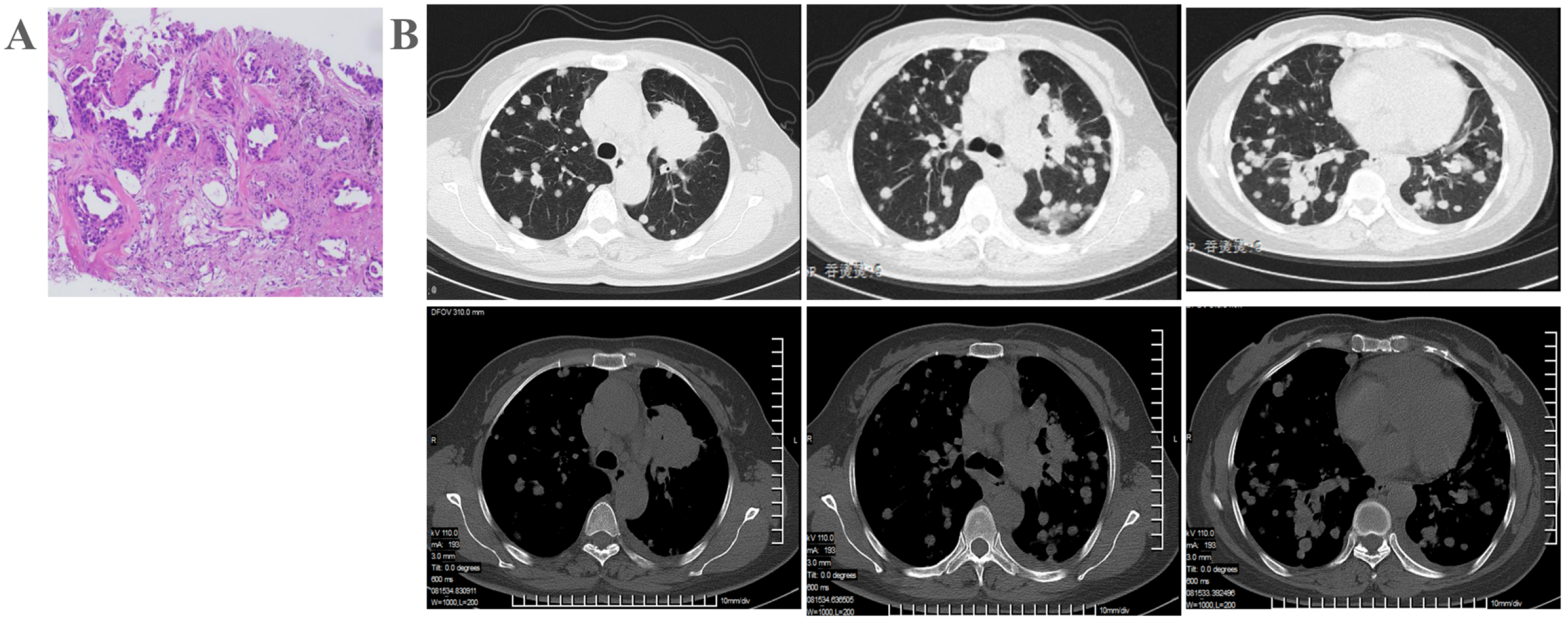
Patient examination before treatment. (A) Chest CT indicates left upper lung cancer with a high likelihood of bilateral lung metastasis, and multiple enlarged mediastinal lymph nodes. (B) Pathological biopsy confirms lung adenocarcinoma.
